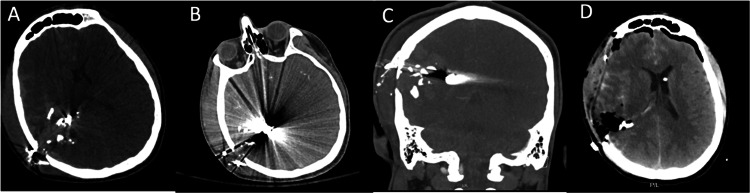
Preoperative axial and coronal CTH and postoperative axial CTH A) Axial CTH with a subdural window, B) axial CTH with the foreign body artifact, C) coronal CTH with a subdural window all demonstrating right parietal penetrating gunshot trajectory with associated subdural hematoma and multiple comminuted skull fragments with intraparenchymal hemorrhage in the parietal cortex with retained bullet fragment foreign bodies, D) postoperative axial CTH, showing improvement in the midline shift and superficial hematoma evacuation and left frontal interval EVD placement with the catheter tip terminating in the left lateral ventricle. CTH: computed tomography of the head
